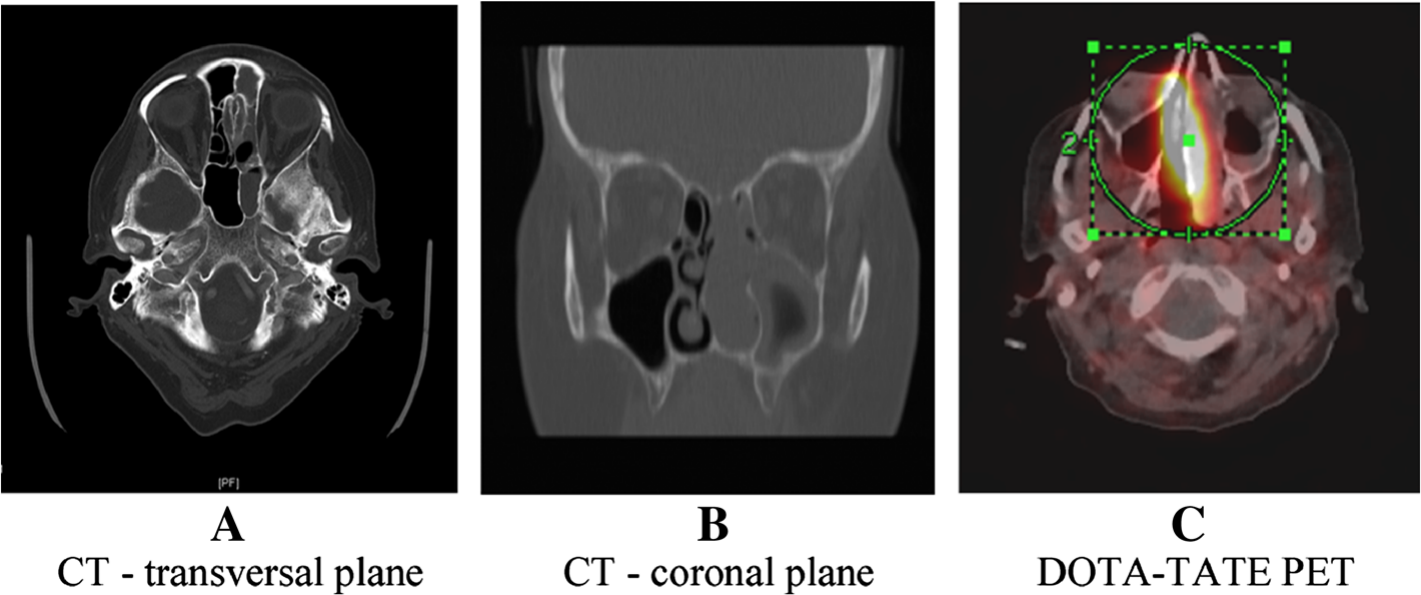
Computed tomography and [68Ga-DOTA]-TATE-Positron-Emission-Tomography of an ACTH-producing tumor localised in the left paranasal sinus. A: Computed tomography (A: coronal, B: transversal) and [^68^Ga-DOTA]-TATE-Positron-Emission-Tomography coregistered with computed tomography (C), displaying a tumorous lesion completely obliterating the left paranasal sinus.
